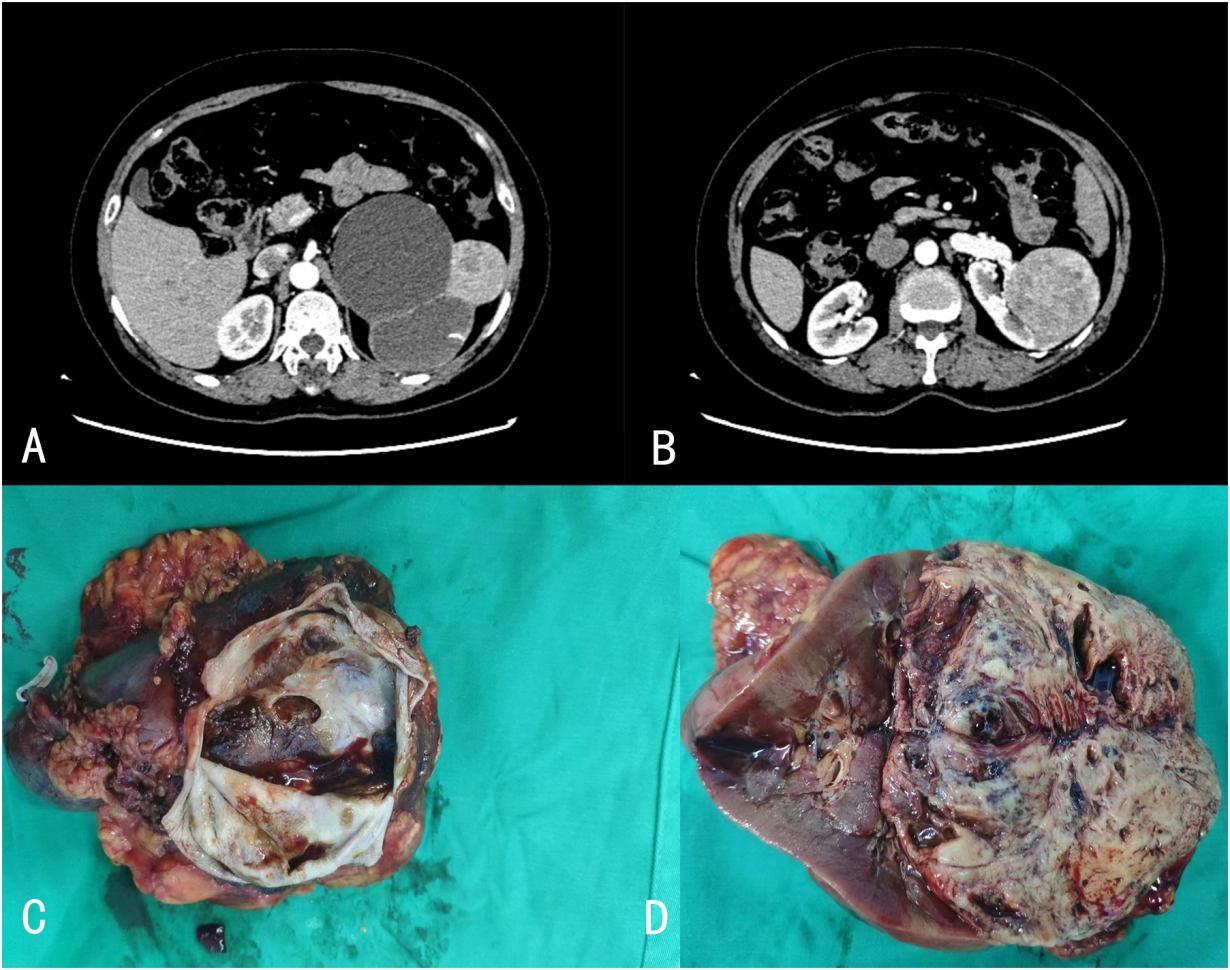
(A, B) Enhanced CT revealed a heterogeneous soft tissue density mass in the left kidney, with significant heterogeneous enhancement during the arterial phase, decreased density during the venous and pyelographic phases, and non-enhanced areas within, measuring approximately 6.9 × 7.1 cm in size. A low-density round lesion with Bosniak classification of IIF was also observed in the same kidney. (C, D) The postoperative pathology revealed the presence of a mass in the upper pole of the kidney, measuring 10 cm × 9.5 cm × 6.5 cm in size, with a cystic-solid appearance on cross-section. The solid area accounted for 60% of the mass, with a pale yellow, solid, and soft texture. Two cystic cavities, measuring 6 cm and 8 cm in diameter, were observed within the cystic area.
